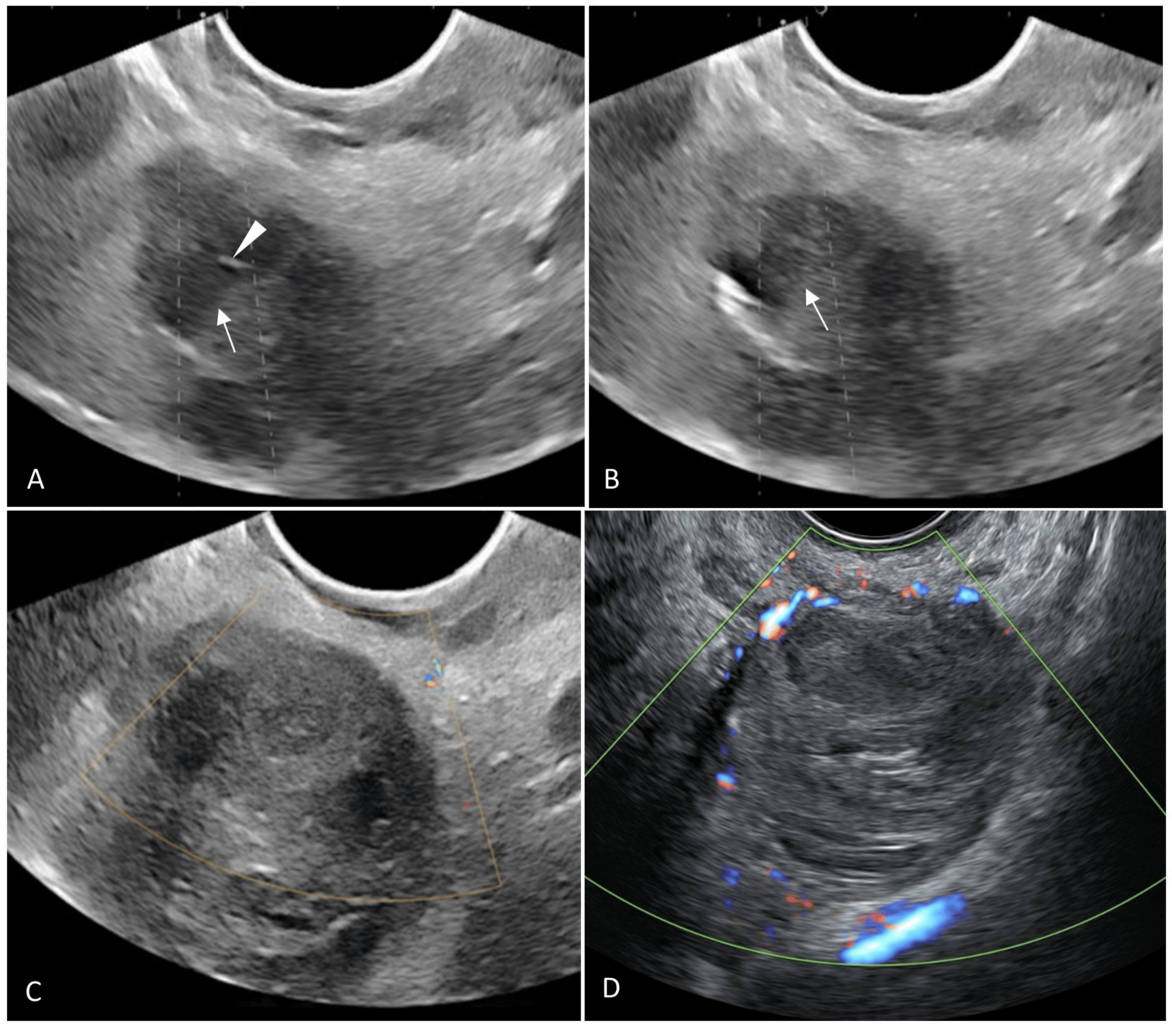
TVS-guided thrombin injection (A) TVS image showing a 22G Chiba needle (arrowhead) being advanced into the pseudoaneurysm (arrow). (B) TVS image showing echogenic thrombus (arrow) formed within the pseudoaneurysm following thrombin injection. (C) Post-thrombin injection color Doppler TVS showing no residual filling of the pseudoaneurysm. (D) Follow-up color Doppler TVS performed after one month showing persistent occlusion of the pseudoaneurysm with reduction in the size of the hematoma TVS: transvaginal sonography
